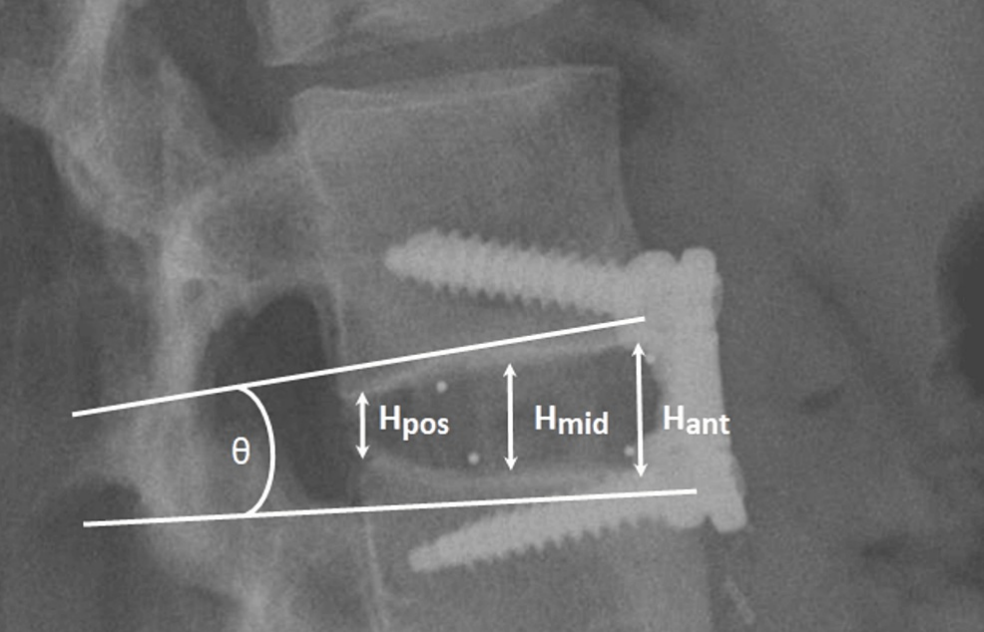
Radiographic Measurement of Intervertebral Disc Space Height and Segmental Angulation. Anterior disc space height (Hant) was measured as the vertical distance between the anterior inferior vertebral endplate of the superior vertebral body and the anterior superior vertebral endplate of the inferior vertebral body. Middle disc space height (Hmid) and posterior disc space height (Hpos) were similarly measured using the middle and posterior vertebral endplates. Segmental angulation was measured as the degree of angulation (θ) formed between the endplates of the superior and inferior vertebral bodies.
